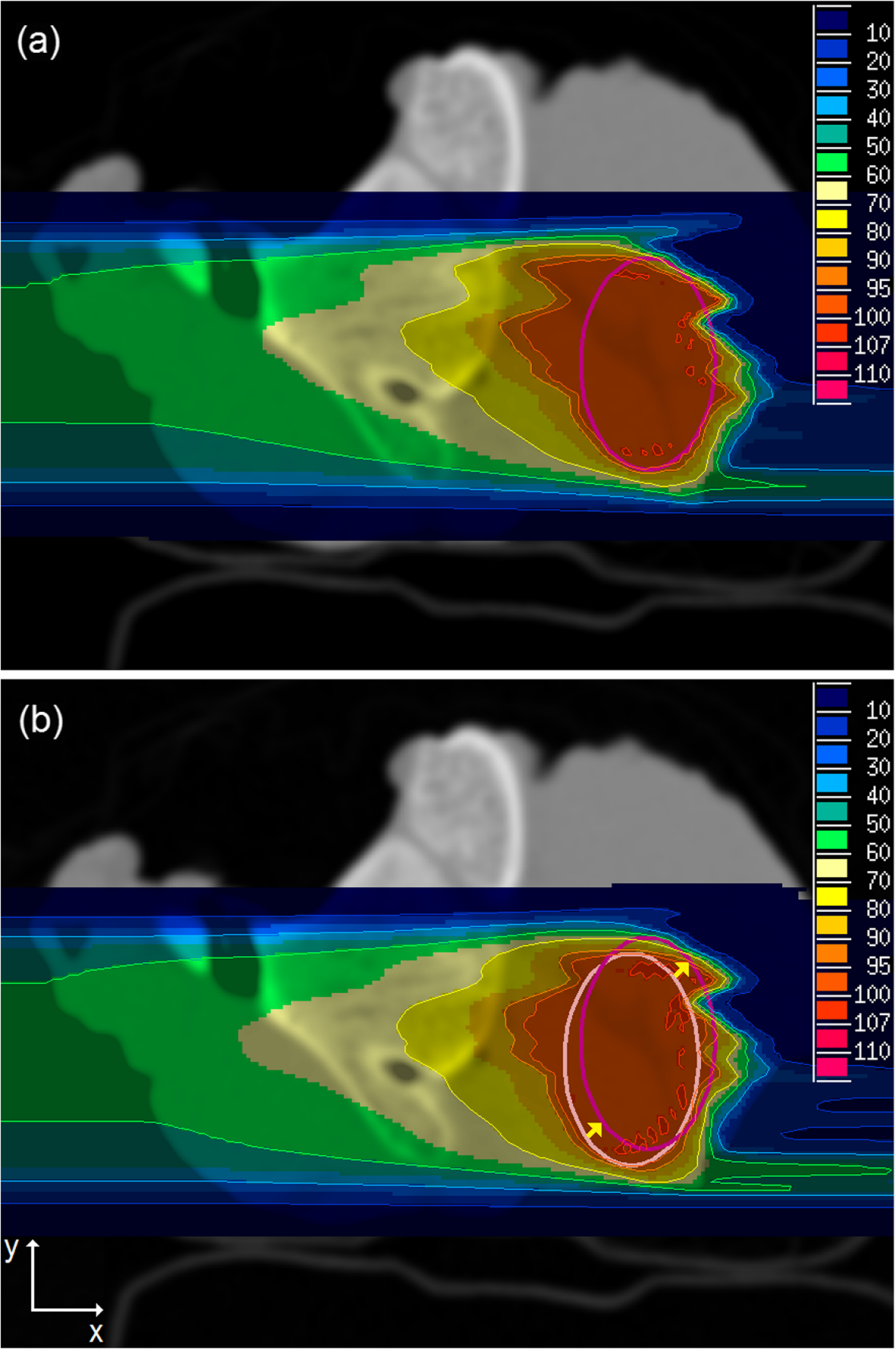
Carbon ion plans of sample 2 with shifted PTV. The old PTV (light magenta) was shifted 2.0 mm in *x*- and 2.0 mm in *y*-direction to a new position (dark magenta): (a) adapted plan optimized on p CT after PTV shift and recalculated on r CT, (b) optimized on r CT before PTV shift and recalculated on r CT after PTV shift without plan adaptation. The unit of color scale is percent of the prescribed physical dose.
